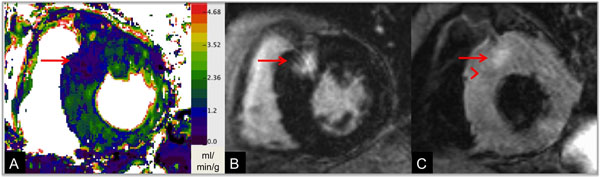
70-year-old patient with HCM and an LV outflow gradient at rest of 28 mmHg. Intense, well-defined MDE in the septum at the anterior right ventricular insertion (B) with corresponding hypo-perfusion (relative MBF = 56%, A). T2-weighted imaging depicts high signal, indicating edema (arrow), and adjacent low signal (arrow head, C).
